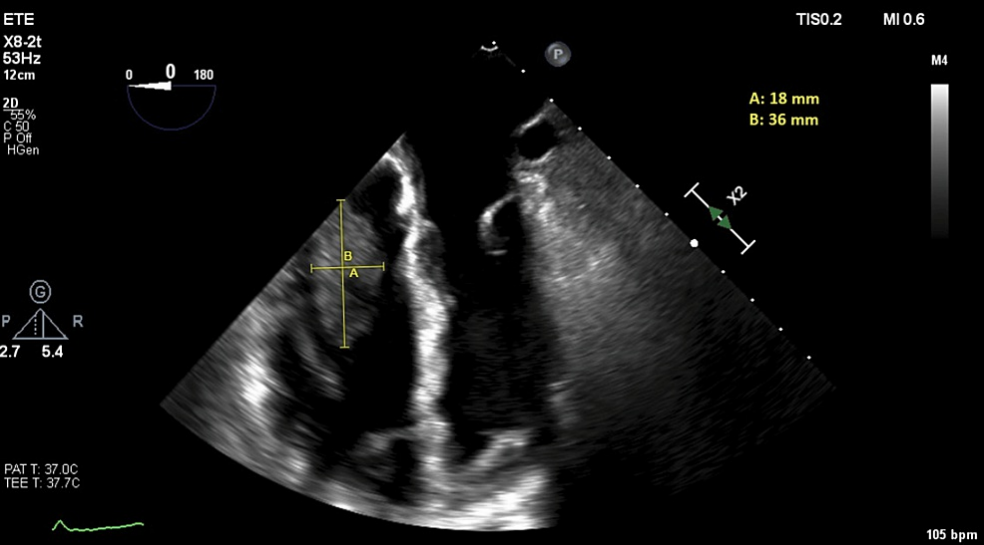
Transoesophageal echocardiogram The image shows the large irregular hypermobile right atrium mass (18 x 36 mm) adjacent to an indwelling catheter protruding through the tricuspid valve.
